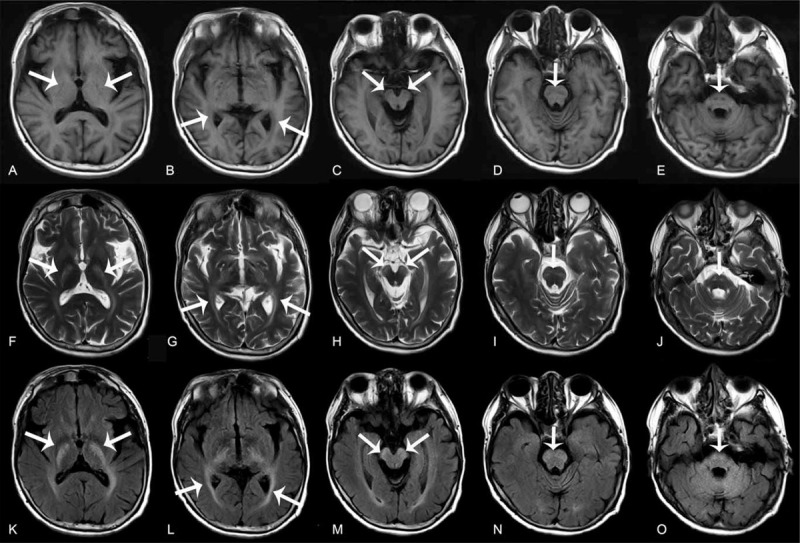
Brain abnormality detected by MRI in the patient. (A) to (E) Axial T1W, (F) to (J) T2W, and (K) to (O) FLAIR images show symmetric hyperintensities in the posterior limbs of internal capsules, paraventricular white matter, cerebral peduncles and anterior region of the pons on T2W and FLAIR images (arrows) with corresponding hypointensities on T1W images, which are along corticospinal tracts. FLAIR = fluid-attenuated inversion recover, T1W = T1-weighted, T2W = T2-weighted.
